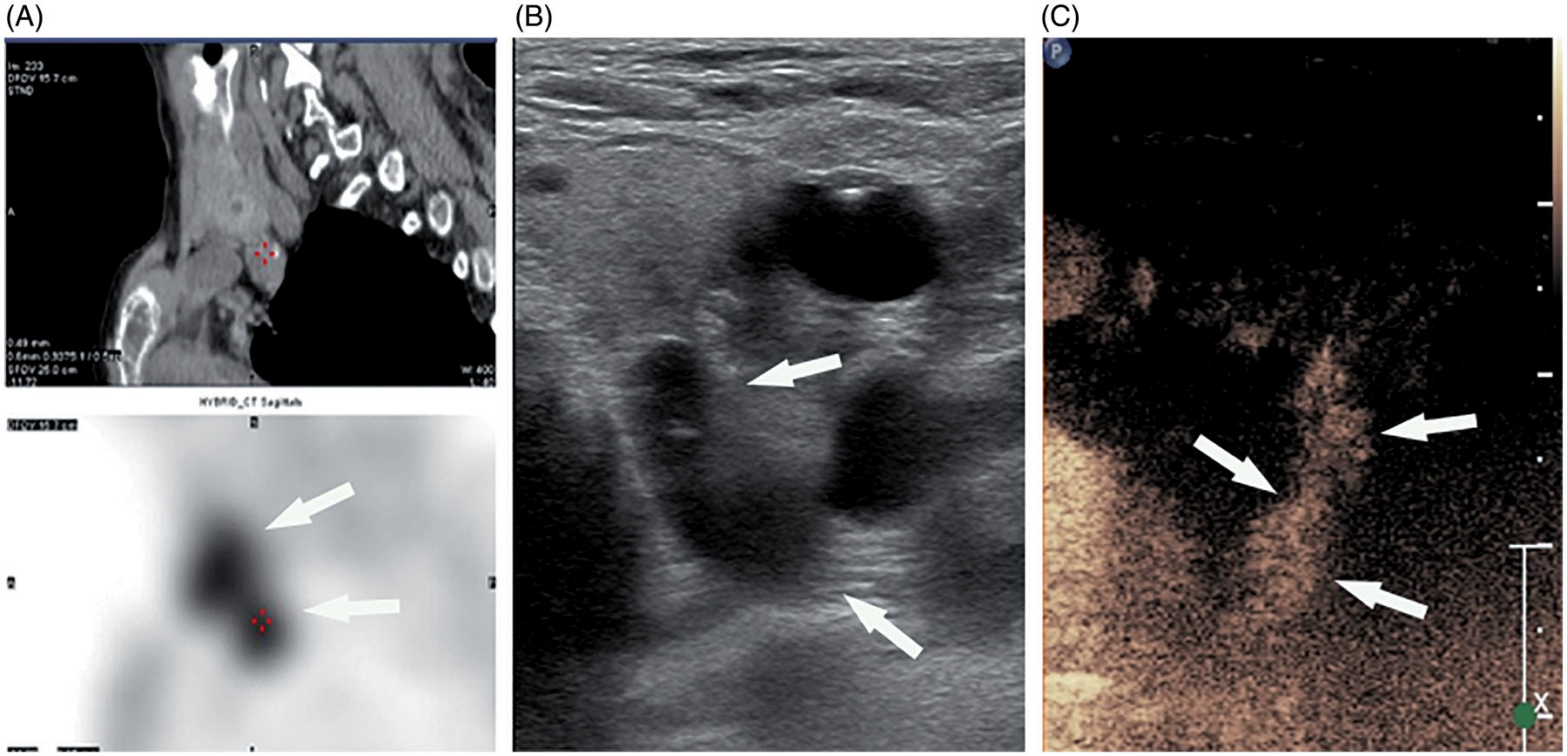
(A) 99mTc-MIBI shows that the nodule has concentration of radioactivity (arrow) in the late phase of the 99mTc-sestamibi sequence. (B) Axial US image shows SHPT hypoechoic nodule boundary behind the lateral lobe of the thyroid and between the carotid artery and trachea before RFA (arrow). (C) After the ablation, contrast-enhanced ultrasonography (CEUS) showed little enhancement of the glands near the trachea (arrow).
